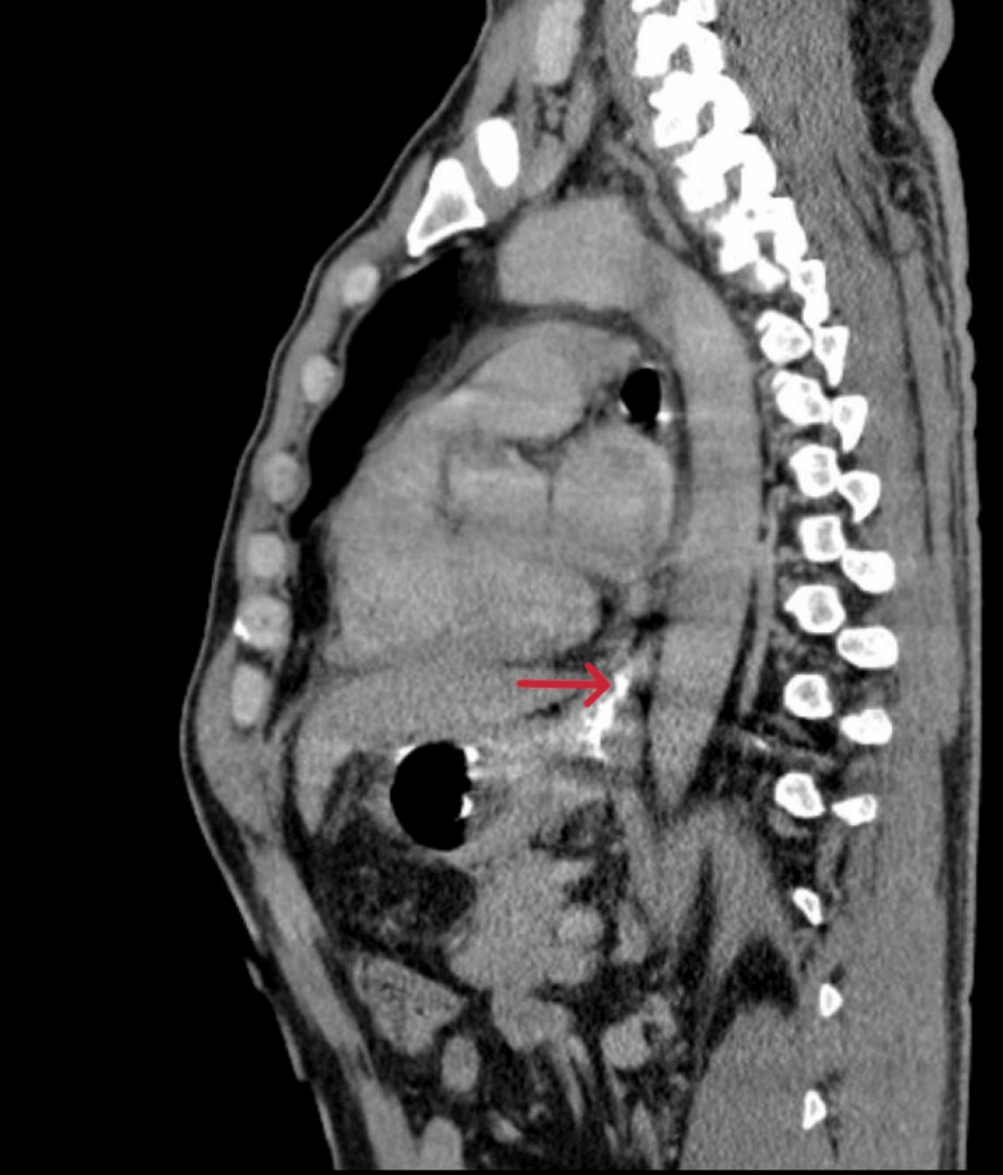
Contrast CT showing lower esophageal stricture
